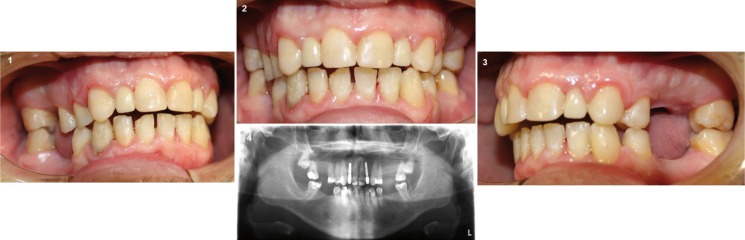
Follow up after 1 year. (1) Right lateral intraoral photography, (2) Frontal intraoral photography, (3) Left lateral intraoral photography and (4) Orthopantomography.
